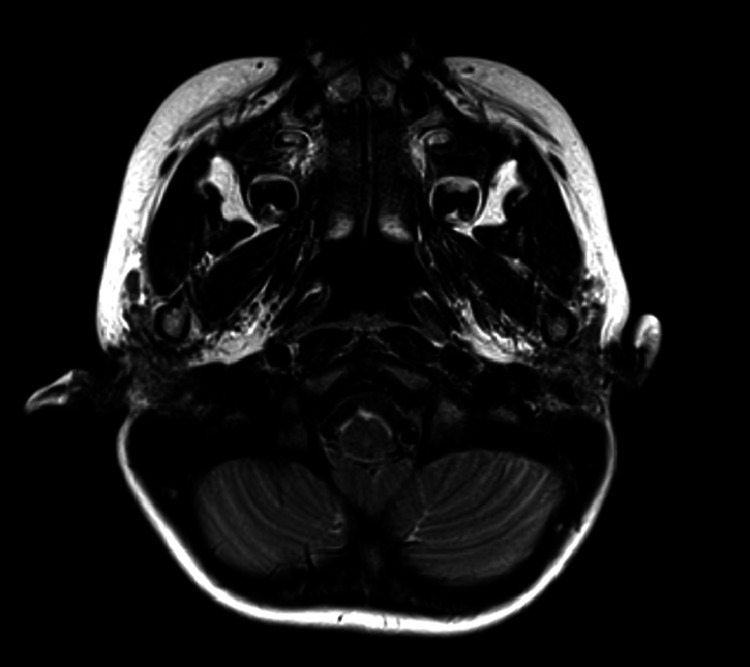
Imaging of Case 2: temporal MRI (T2-weighted image) MRI, magnetic resonance imaging.
